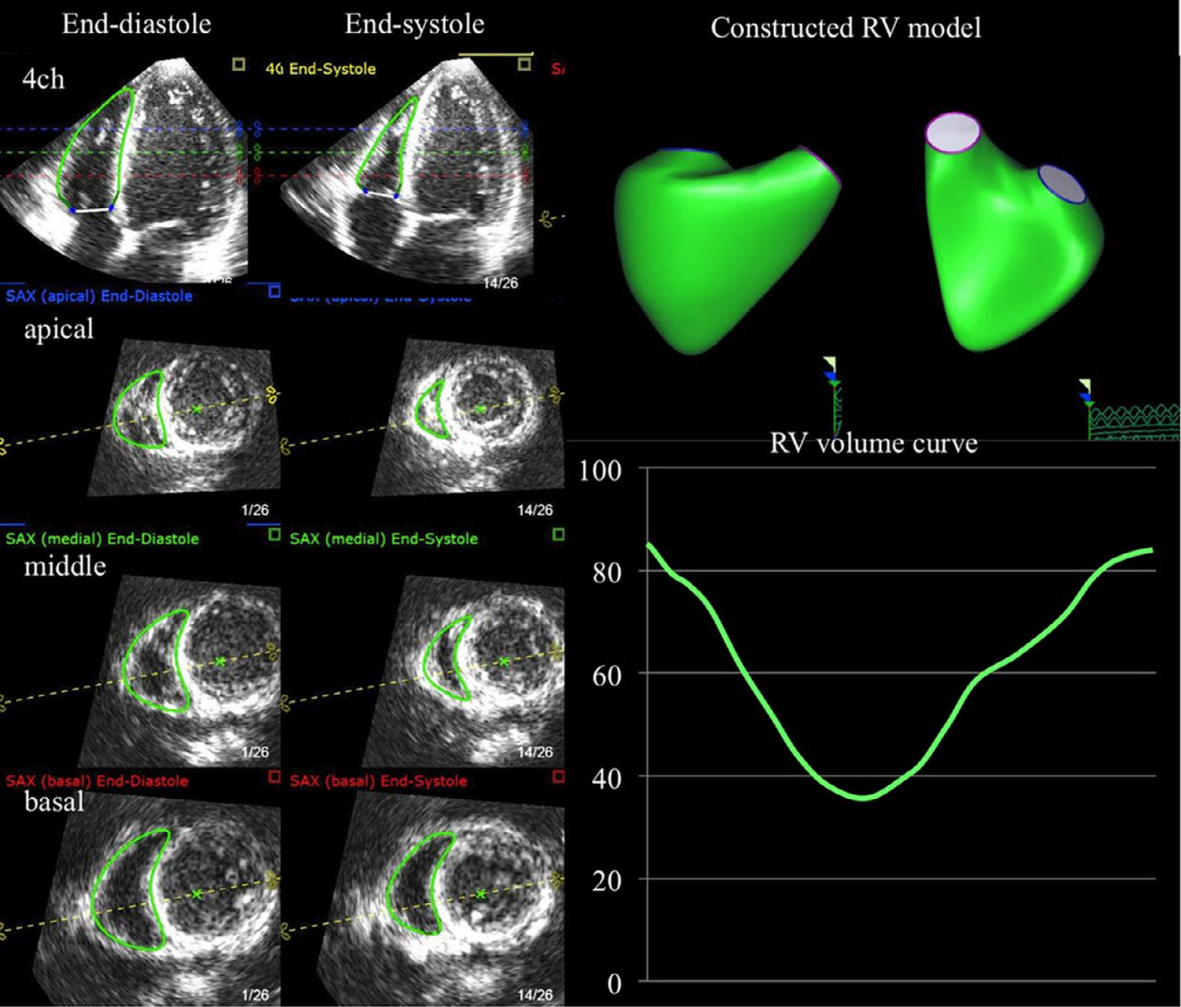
3D Echocardiographic assessment of right ventricular volumes and function.
